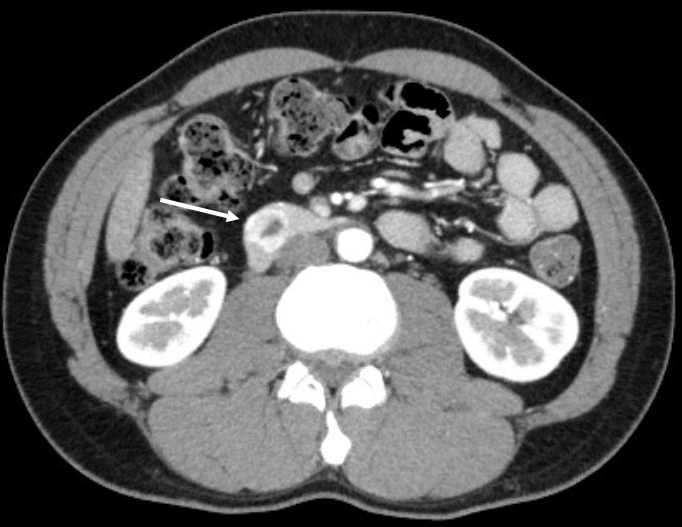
(Case 1) Contrast-enhanced computed tomography showed a heterogeneous tumor in the second portion of the duodenum (arrow).
